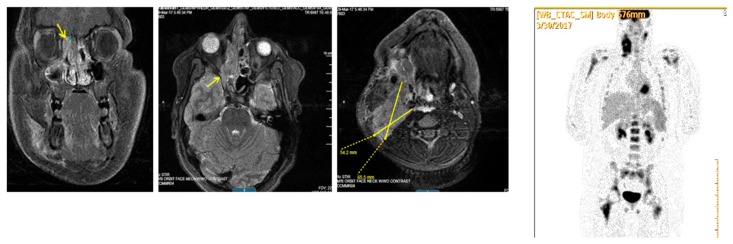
MRI T-1 weighted image of 13 y/o patient with Stage IV parameningeal alveolar RMS. (Left) image shows in a coronal plane a heterogeneous mass centered within the right ethmoid air cells (arrow) and superior nasal cavity mildly displacing the orbit. (Right) image—axial cut shows right neck lymphadenopathy shown as well with high uptake in PET scan image at the far right.
